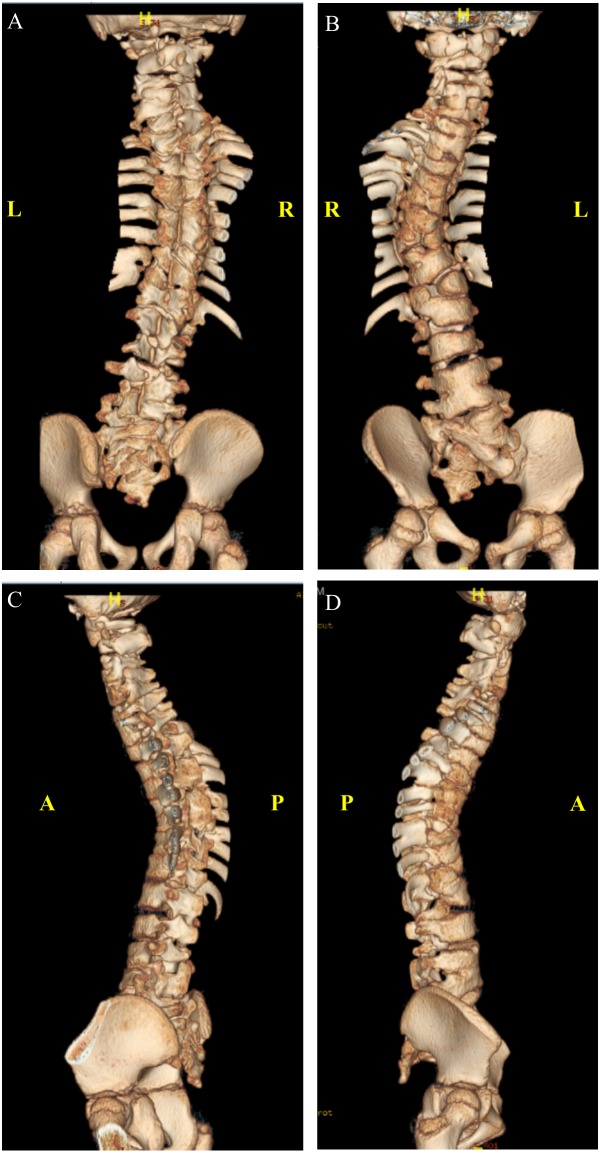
Three-dimensional computed tomography (CT) scan. (A) Anterior. (B) Posterior. (C) Left lateral. (D) Right lateral view.
